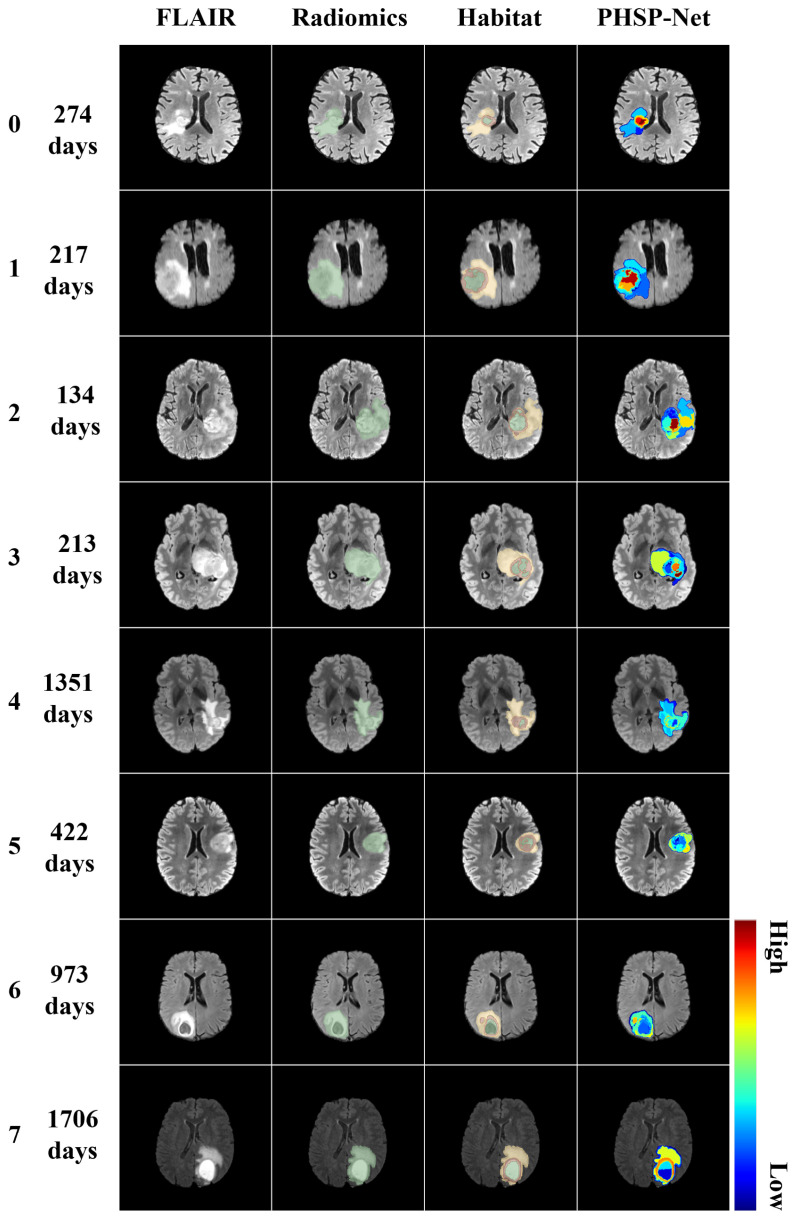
From left to right, the figure shows the FLAIR image, followed by the 2D visualizations of the ROIs for the radiomics, habitat imaging and PHSP-Net models. A color jitter scheme was applied to visually represent the risk of OS across different habitat and radiomics features in patients. Red tones denote higher risk. Patients with survival less than one year are labeled with values 0–3, whereas those with survival times exceeding one year are labeled with values 4–7. Each number from 0 to 7 corresponds to a patient, along with their specific OS time.
